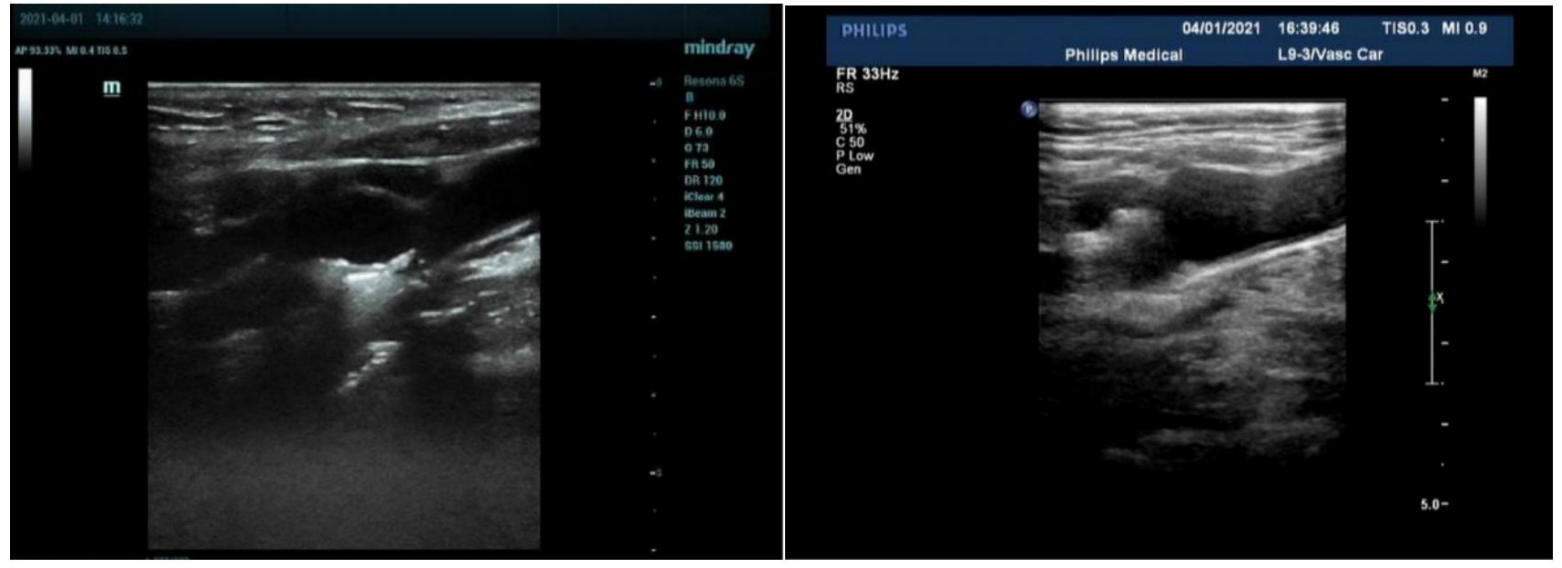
Original ultrasound images of carotid plaque saved in DICOM
